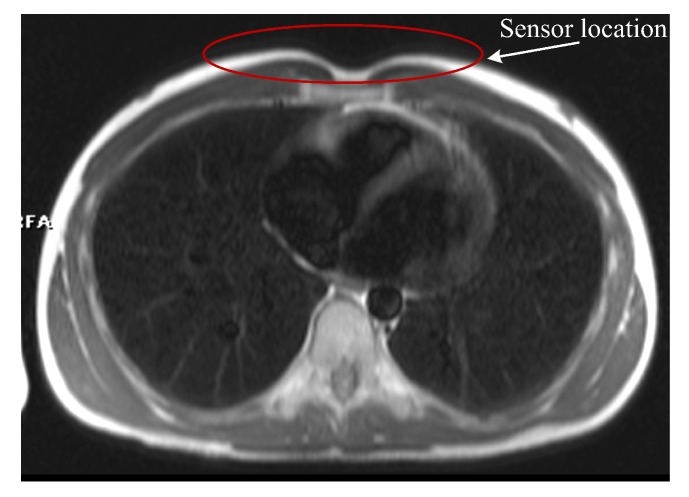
A sample MRI image (axial view) showing where the FBG sensor was positioned: A GE T2-weighted image (400 × 400 pixels).
